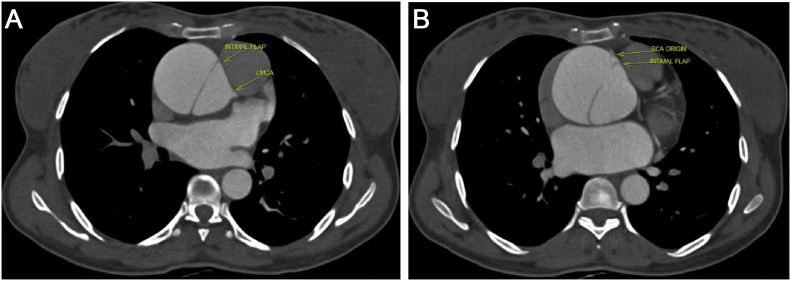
A and B: Axial Multiplanar Reconstruction (MPR) of the 45% phase of the gated CT Aortogram study showing the relationship of the intimal flap with the right coronary artery (RCA) and the left main coronary artery (LMCA).
